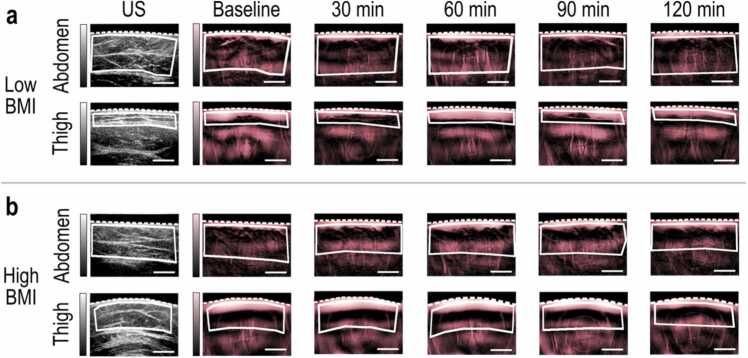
Exemplary MSOT image series of SAT hemodynamics at different time points during the oral glucose. (a) Representative ultrasound (US) and 800 nm-MSOT frames in the abdominal and thigh regions for an individual of low BMI (body mass index). (b) Representative US and 800 nm-MSOT frames in the abdominal and thigh regions for an individual of high BMI. White dashed line: skin, white continuous line: subcutaneous adipose tissue (SAT) region, Scale bars: 1 cm.
